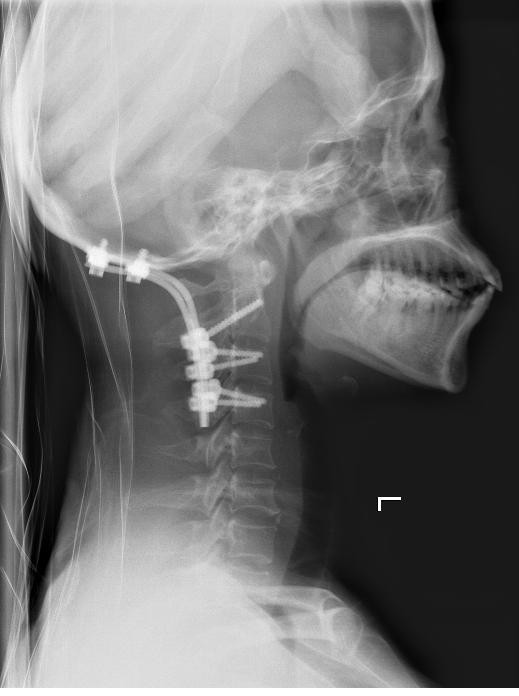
Lateral X-ray postoperative image.
